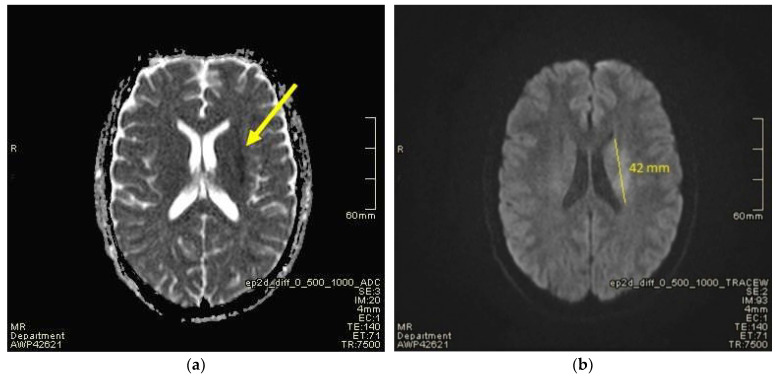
Ischemic strokes involving the corona radiata and lenticular nuclei on the left side. Measurement of AP (anteroposterior) dimension was about 42 mm—changes visible only in the ADC (a) and DWI (b) sequence. Yellow arrow (a) and measurement line (b) represent described changes.
